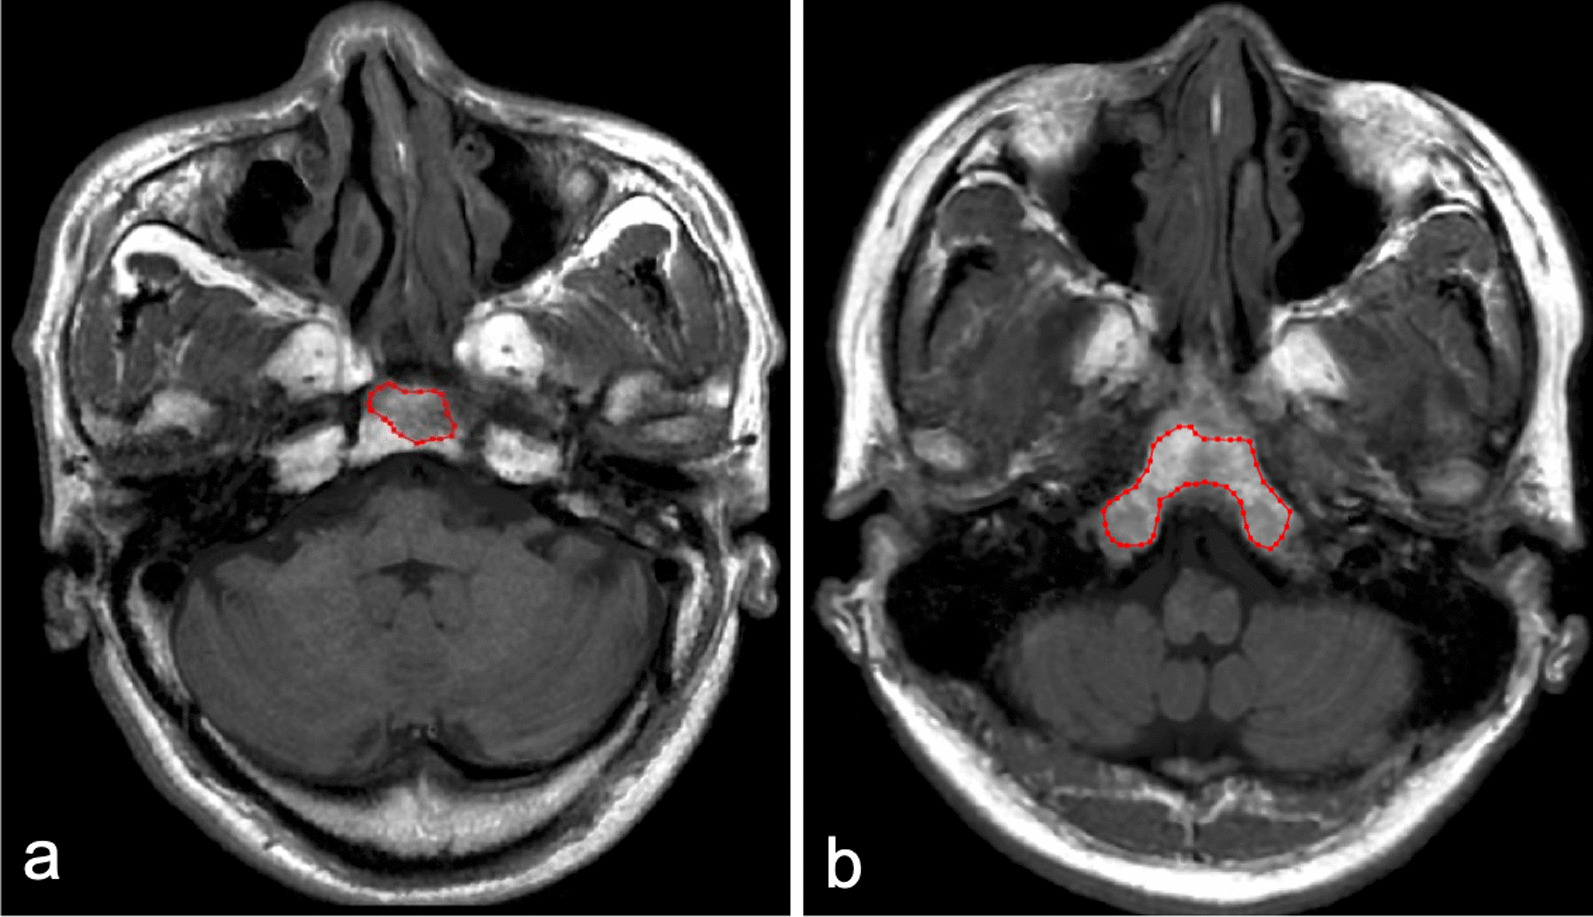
Representative images of OC invasion and OC non-invasion. Invaded OC in 56-year-old NPC patient (a) shows low signal on axial T1WI of SyMRI, which shows similar signal characteristics with non-invaded OC in 38-year-old volunteer (b). VOI was manually delineated along the border of invaded OC and non-invaded OC on axial T1WI of SyMRI. OC, occipital clivus; NPC, nasopharyngeal carcinoma; T1WI, T-weighted image; SyMRI, synthetic magnetic resonance imaging; VOI, volume of interest
